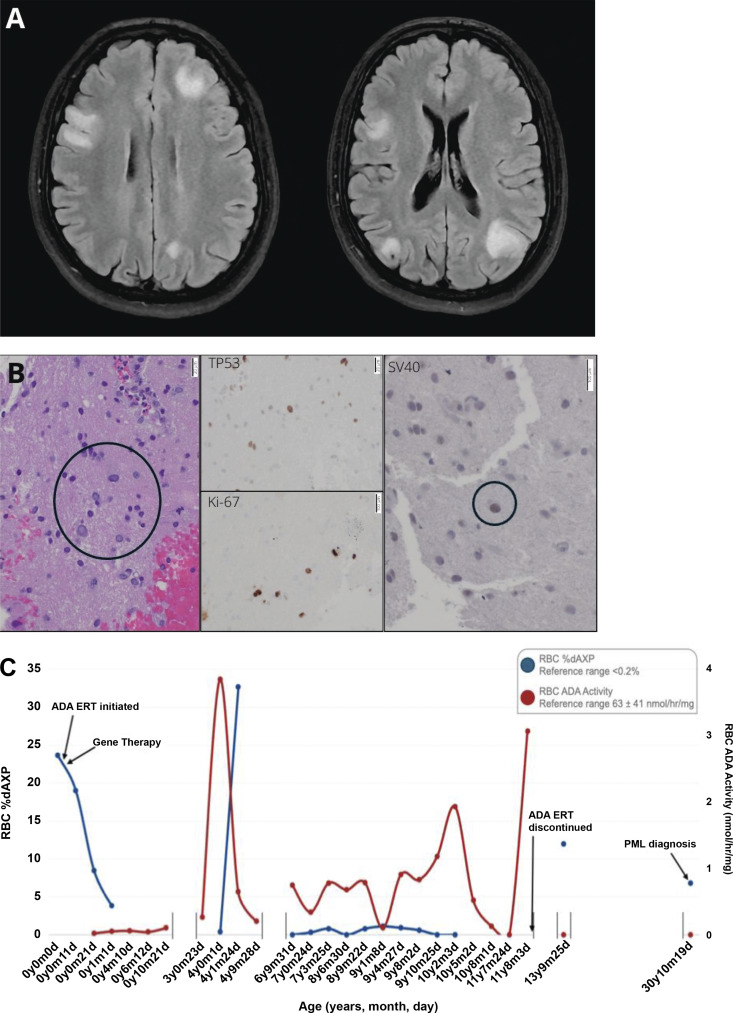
Brain MRI, brain biopsy, and longitudinal biochemical monitoring in this patient with ADA-SCID who developed progressive multifocal leukoencephalopathy.
